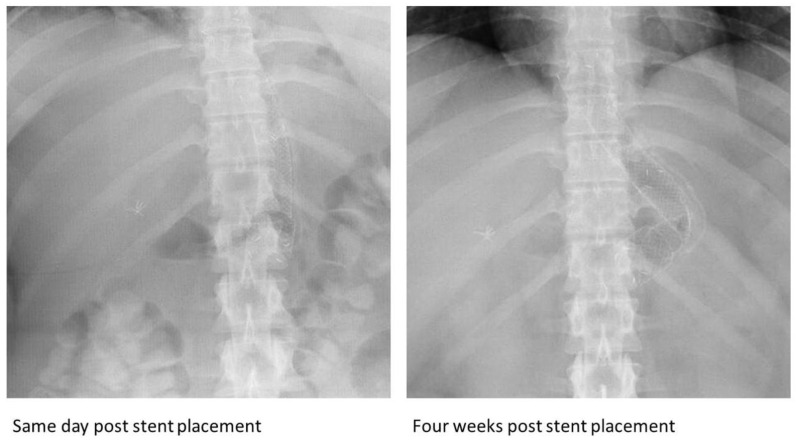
Subsequent radiographs after the placement of the esophageal fully covered self-expandable metal stent in the first case, demonstrating acute angulation and a possible twist at the same location of the complete fracture.
